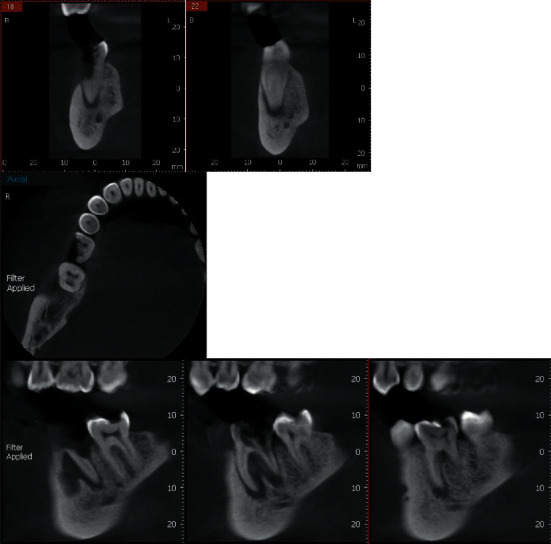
CBCT scanning preoperatively.
